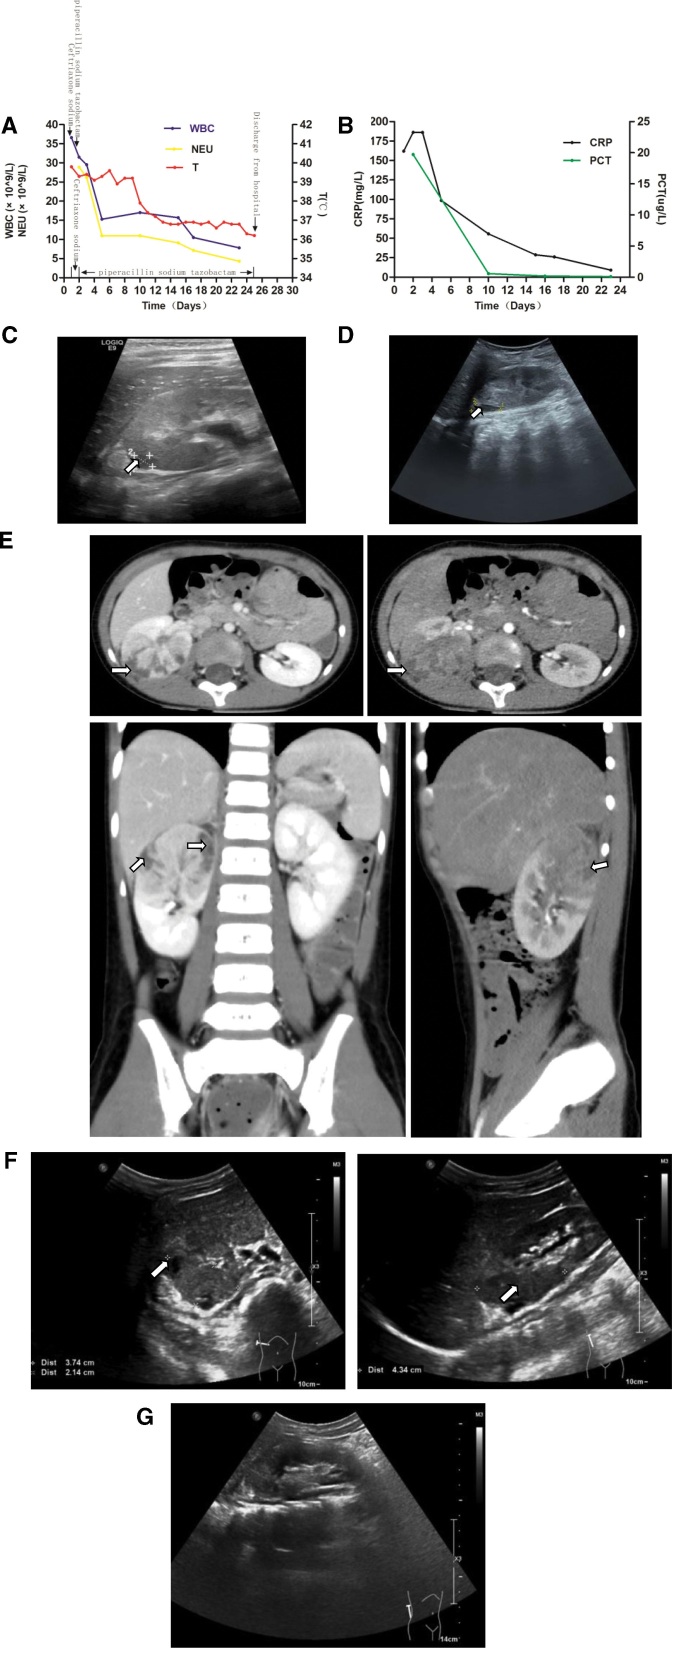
The changes of clinical indexes, kidney B-ultrasound, computed tomography (CT) before, and after treatment in case one. (A) Changes of white blood cell counts, neutrophil counts, and body temperature in case one. The white blood cell counts and neutrophil counts were significantly increased on admission, and the body temperature was repeatedly high, which decreased to normal after anti-infection treatment. (B) Changes of CRP and PCT in case one. The levels of CRP and PCT were significantly increased on admission, and went back to normal after anti-infection treatment. (C, D, F, and G) Renal ultrasonography was performed on the 2nd, 10th, 15th, and 23rd day after admission for case one. The abscesses of the right kidney were measured as 0.94 cm × 0.93 cm, 1.53 cm × 0.82 cm, 3.7 cm × 2.1 cm × 4.3 cm (arrow), and the abscesses were completely subsided on day 23. (E) Enhanced CT scan performed on day 6 of admission showed abnormal enhancement in the upper pole and middle of the right kidney, suggesting pyelonephritis with renal abscess formation (arrow). CRP = C-reactive protein, PCT = procalcitonin.
